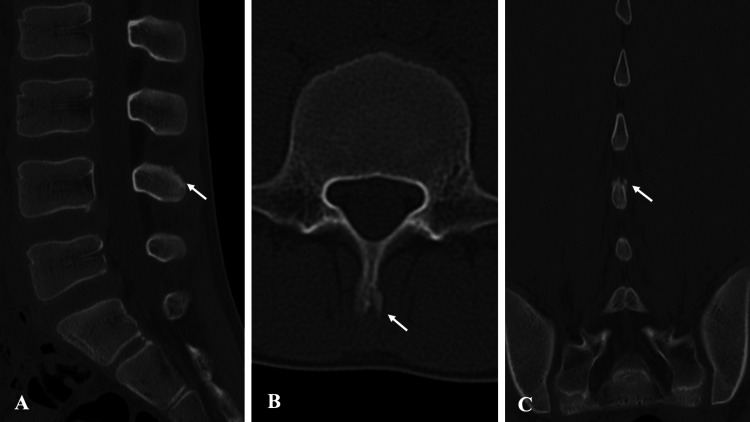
Pre-treatment CT findings in Case 2. CT shows a fracture line at the same location: (A) sagittal view, (B) axial view, and (C) coronal view. CT, computed tomography
